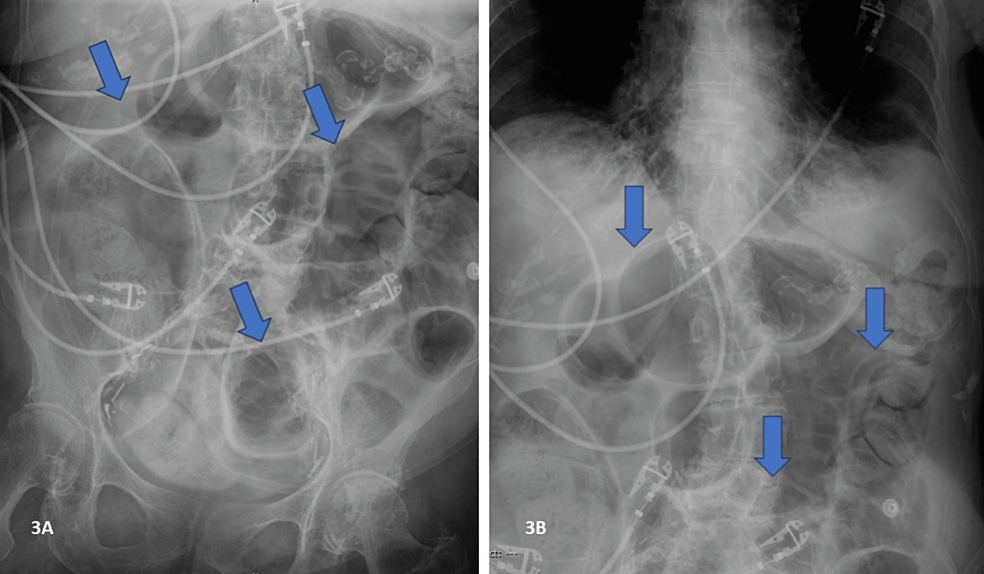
Abdominal radiograph Impression: nonspecific bowel gas pattern. No evidence of intestinal obstruction. No pneumoperitoneum
